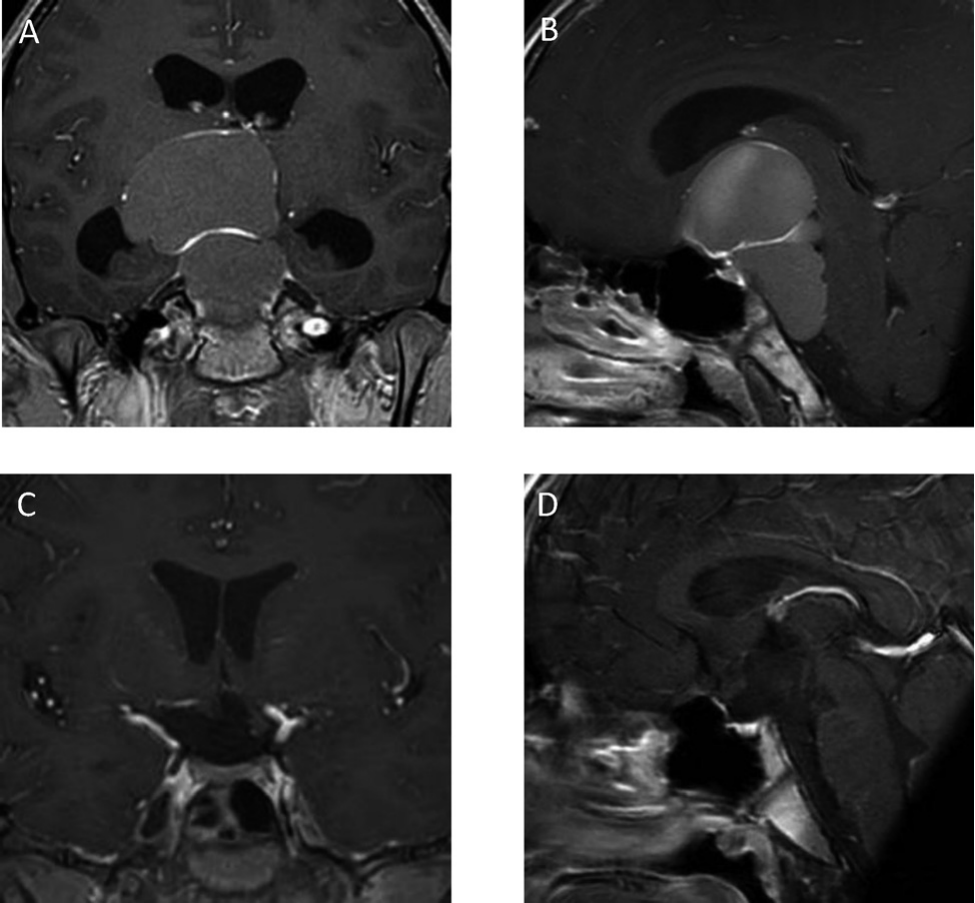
Case 1: A 13-year-old boy. Preoperative coronal (A) and sagittal (B) enhanced T1-weighted magnetic resonance imaging (MRI) demonstrated an expanded cystic tumor that encroached the third ventricle and posterior circulation with hydrocephalus. The tumor was totally removed, with postoperative MRI showing no residual tumor (C, D).
